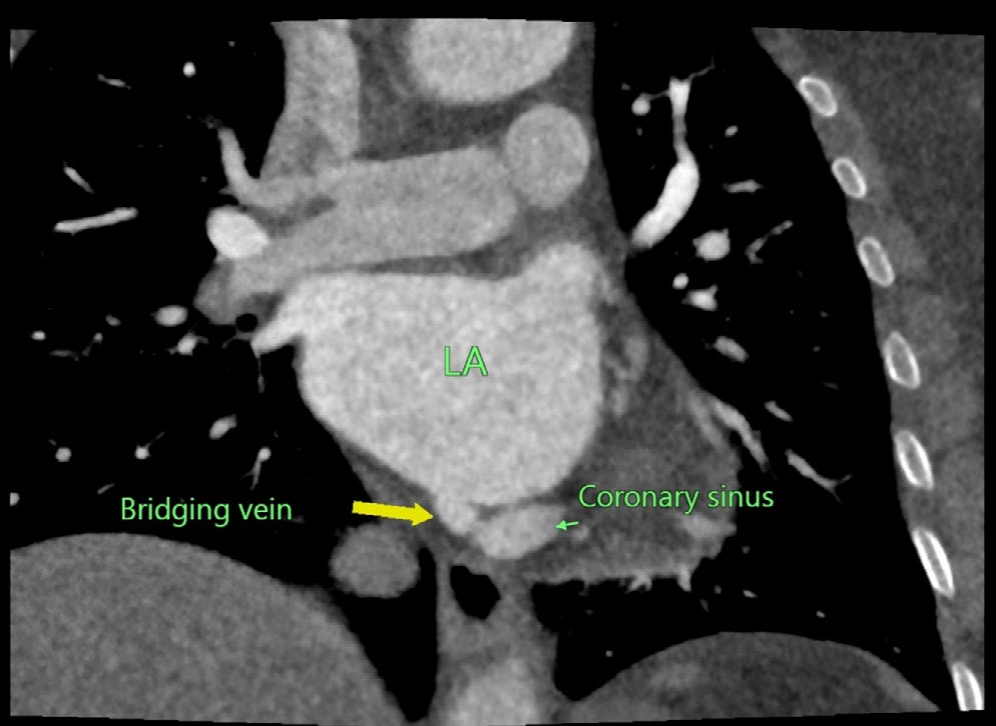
Coronal CT angiogram showing the coronary sinus draining into the left atrium through a bridging vein.
